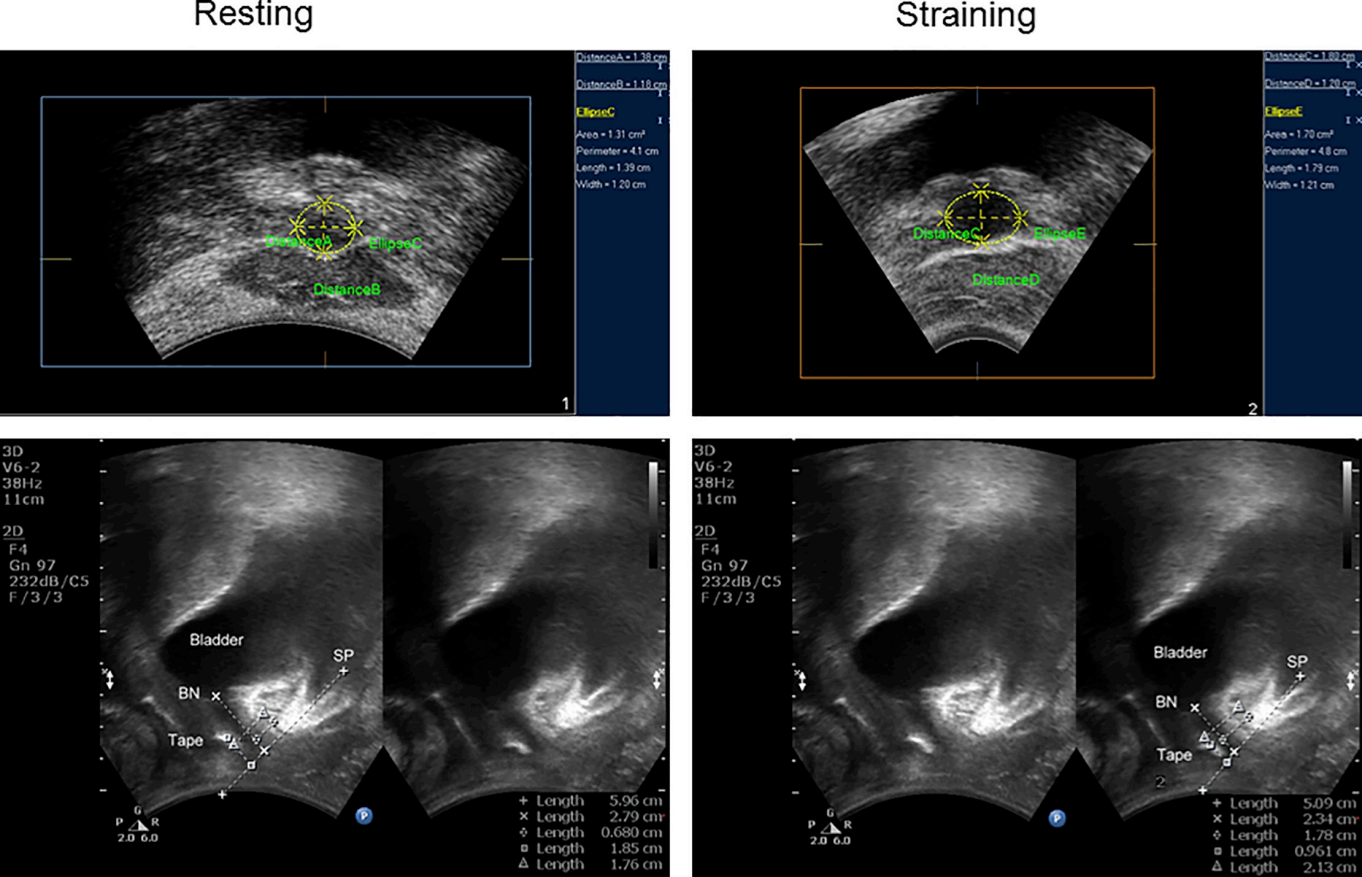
3D and 2D introital ultrasound imaging revealing the lower urinary tract and mid-urethral sling during rest and straining; BN, bladder neck; SP, Symphysis Pubis.
